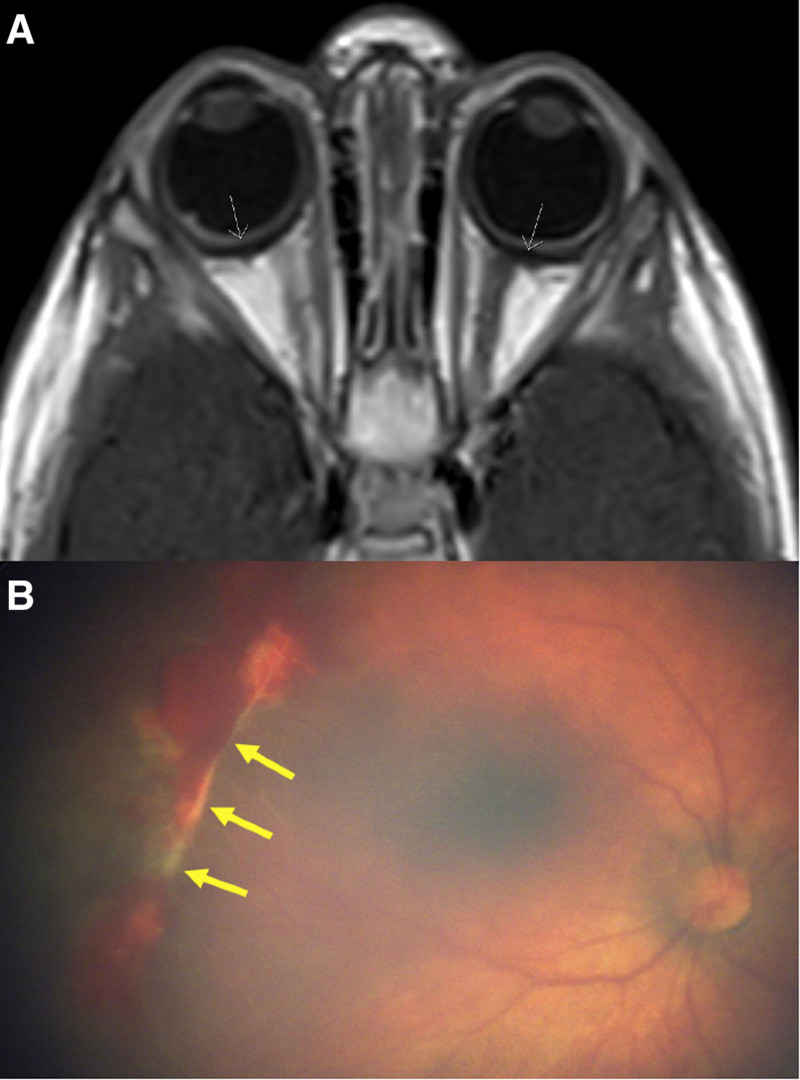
(A) Retinal exudate (arrows) seen on magnetic resonance imaging of the brain. (B) Retinal exudate (arrows) seen on standard ophthalmological exam.
